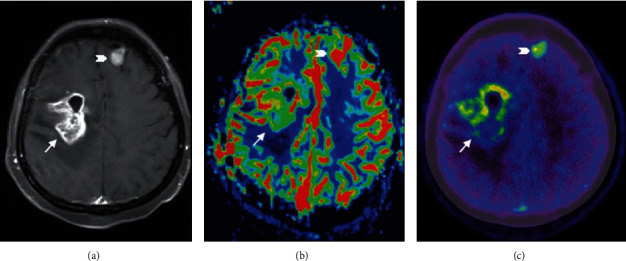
MET PET combined with PWI in recurrent glioblastoma. Contrast-enhanced T1-weighted MR image (a) and a relative cerebral blood volume (CBV) map (b) and a MET PET/CT image (c) for a 41-year-old woman with recurrent glioblastoma. The lesion in the right frontal lobe (arrow) shows enhancement (a) and positive findings on both the rCBV map (b) and MET PET/CT image (c). The lesion localized in the left frontal lobe (chevron) might be a meningioma, which has been stable for several years. Reproduced with permission from Figure 2 of Qiao et al. [58].
